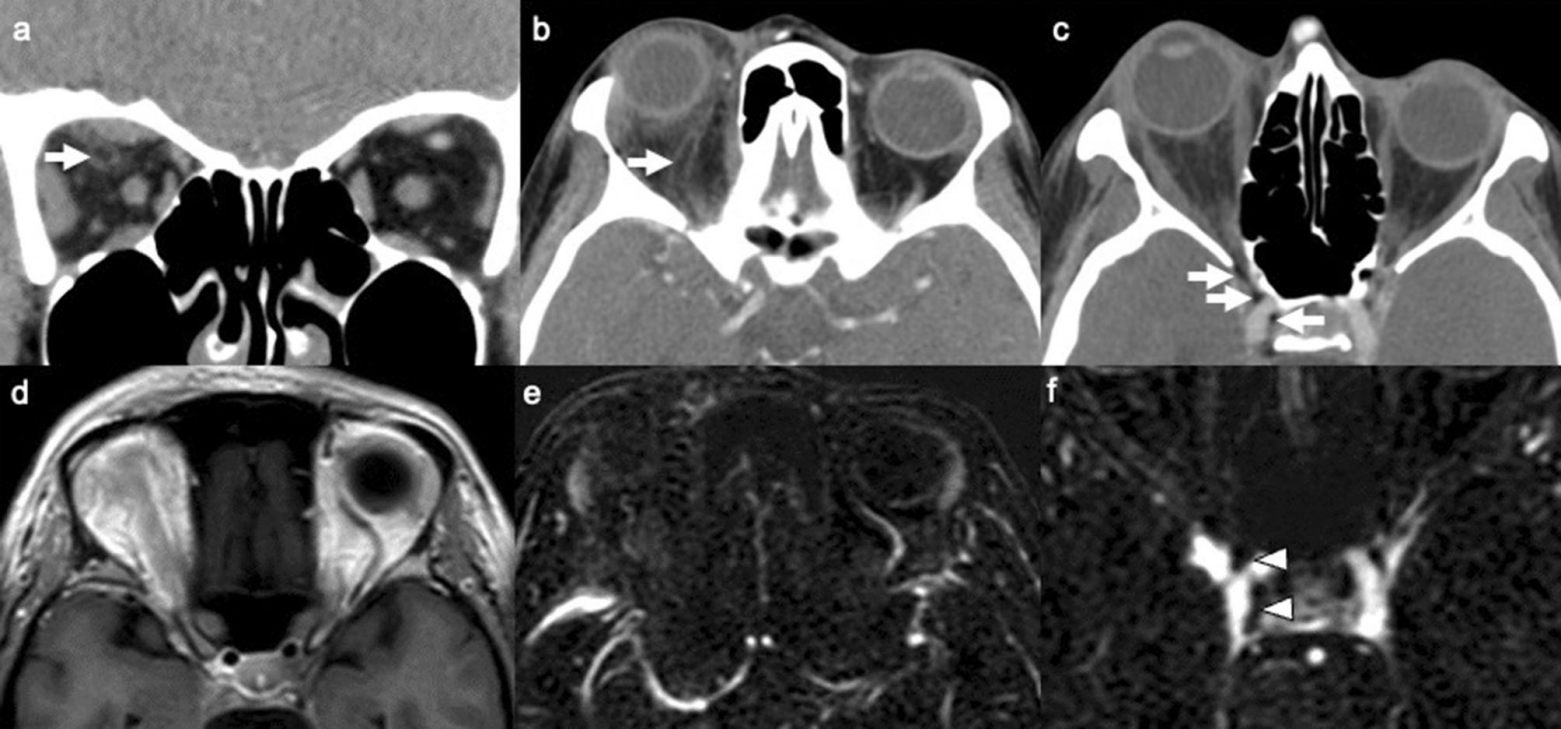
a–c Contrast-enhanced orbit computed tomography showed fat attenuation filling defects in the dilated right superior ophthalmic vein and right cavernous sinus (white arrow). Right proptosis, right retrobulbar fat reticulation, and right extraocular muscle enlargement from orbital congestion were demonstrated. d On T1-weighted images, loss of normal flow void with abnormally increased signal intensity of fat embolism was seen in the right superior ophthalmic vein. e, f Magnetic resonance venography demonstrated no contrast filling in the right superior ophthalmic vein and few small filling defects in the right cavernous sinus (arrowhead)
